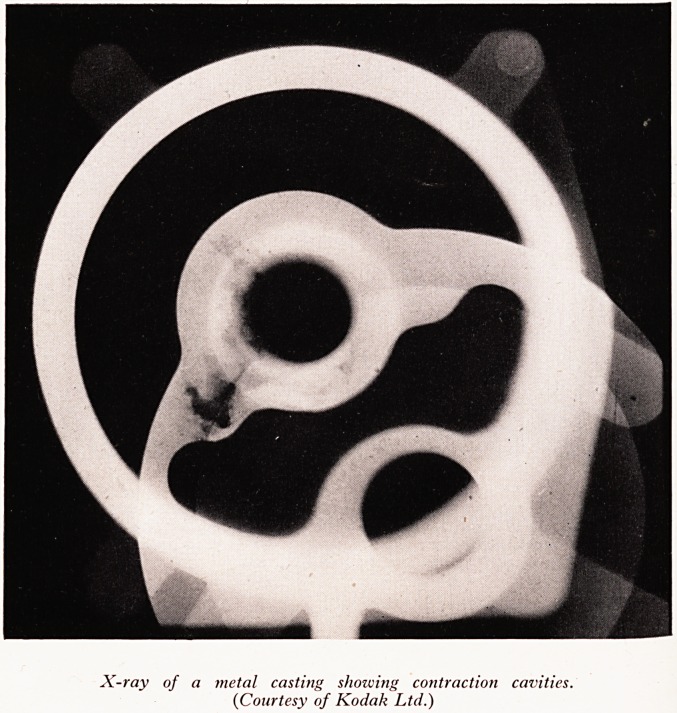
# X-Rays in Everyday Life
